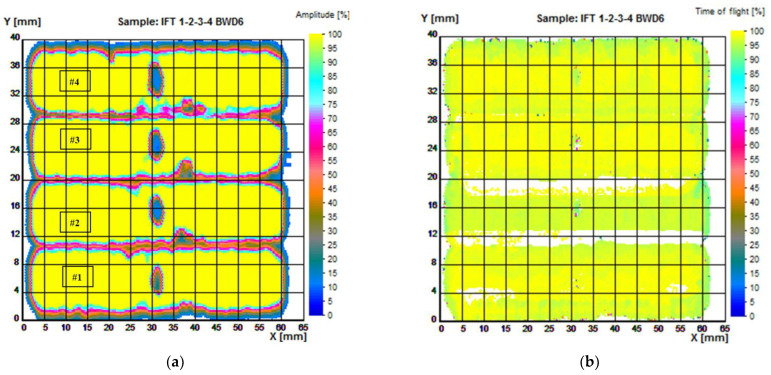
Ultrasonic testing of samples 1 to 4: (a) amplitude image, examination ISA-FE5 on back echo; (b) time-of-flight image, examination ISA-FE5 of back echo.
